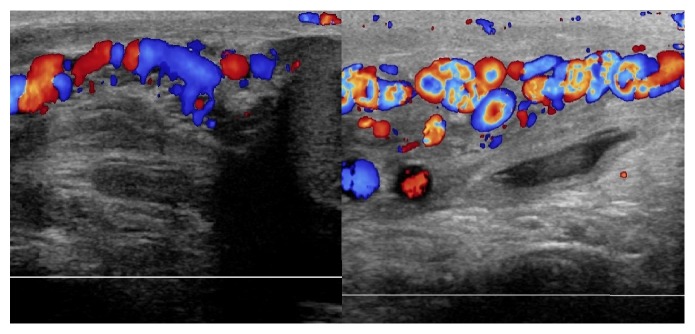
Doppler ultrasonography: dilated paratesticular veins, compatible with the presence of the varicocele. Presence of reflux during Valsalva maneuver.
